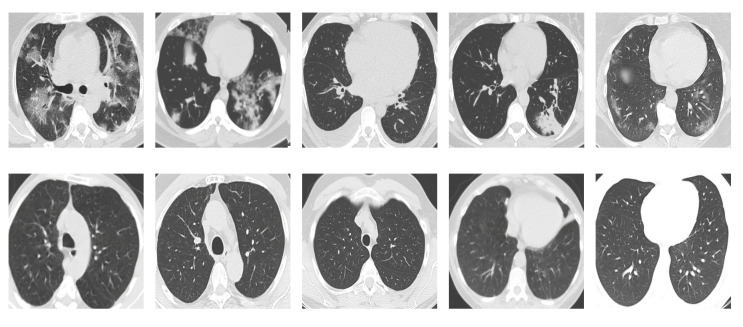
Examples of chest CT scans from the SARS-CoV-2 CT dataset. The first row represents CT scans diagnosed with COVID-19, whereas the second row represents non-COVID-19.
